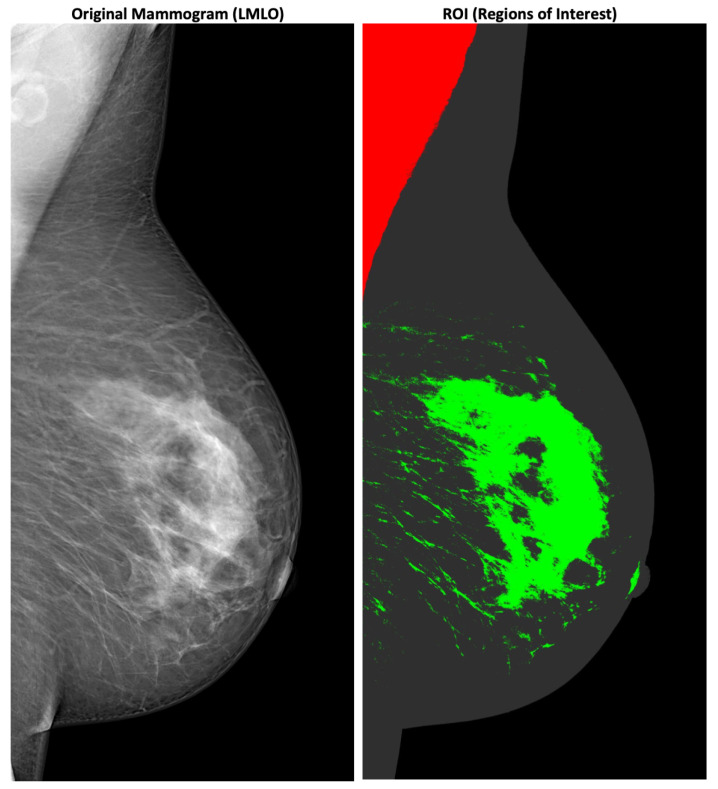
Example of a X-ray mammography with highlighted medical image features [7].
